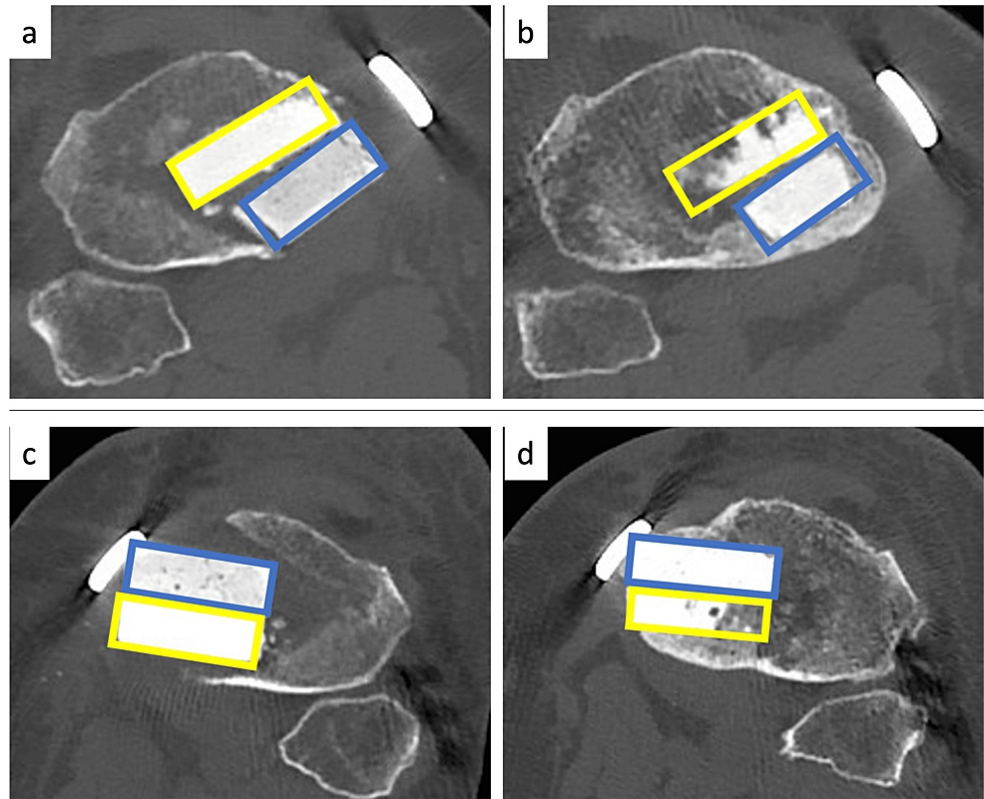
CT findings CT images of the center of the osteotomy plane for Case 1 show the mean CT value (HU) of the area implanted with UDPTCP (yellow rectangle) and the area implanted with SPTCP (blue rectangle). CT images of the U-S knee at one week (a) and last observation (b). CT images of the S-U knee at one week (c) and last observation (d) CT: computed tomography; HU: Hounsfield units; SPTCP: spherical porous β-tricalcium phosphate; UDPTCP: unidirectional porous β-tricalcium phosphate: U-S: UDPTCP was implanted anteriorly and SPTCP was implanted posteriorly; S-U: SPTCP was implanted anteriorly and UDPTCP was implanted posteriorly
